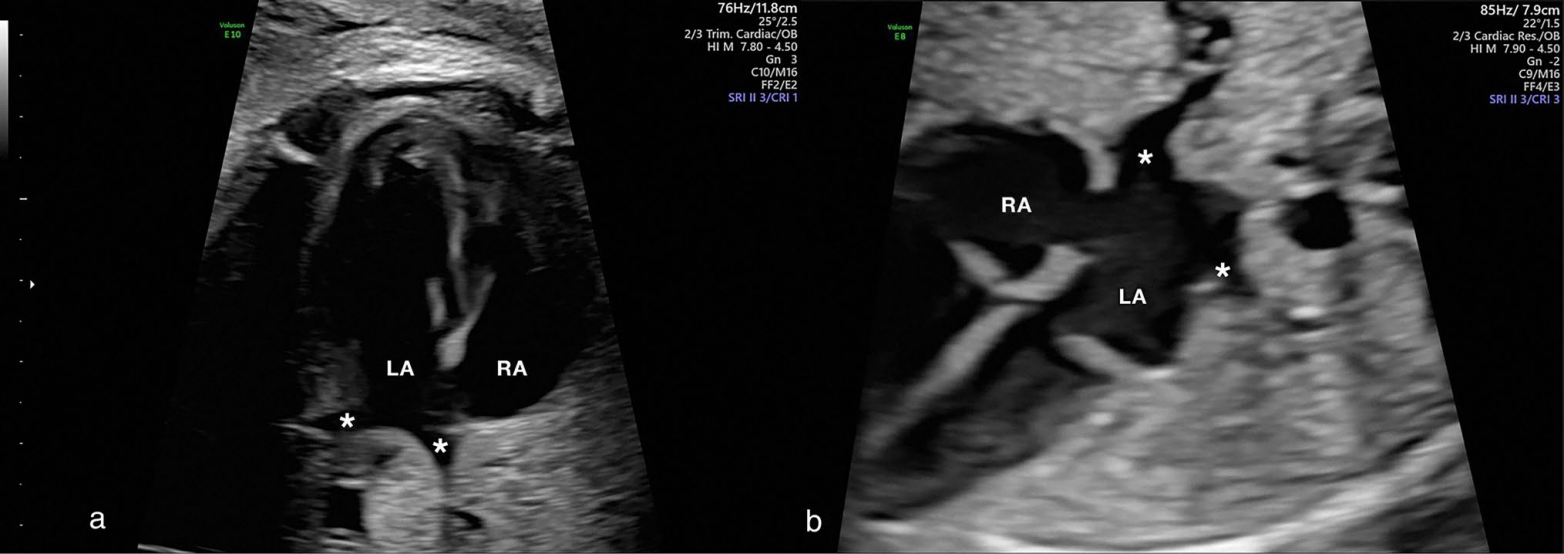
Fetal echocardiography: the ideal plane to visualize the pulmonary veins is the apical four-chamber view (a), showing the connection of both inferior right and left pulmonary veins (*); the pulmonary veins can be visualized in other views, as the basal or 45° four-chamber view (b). *RA* right atrium; *LA* left atrium
